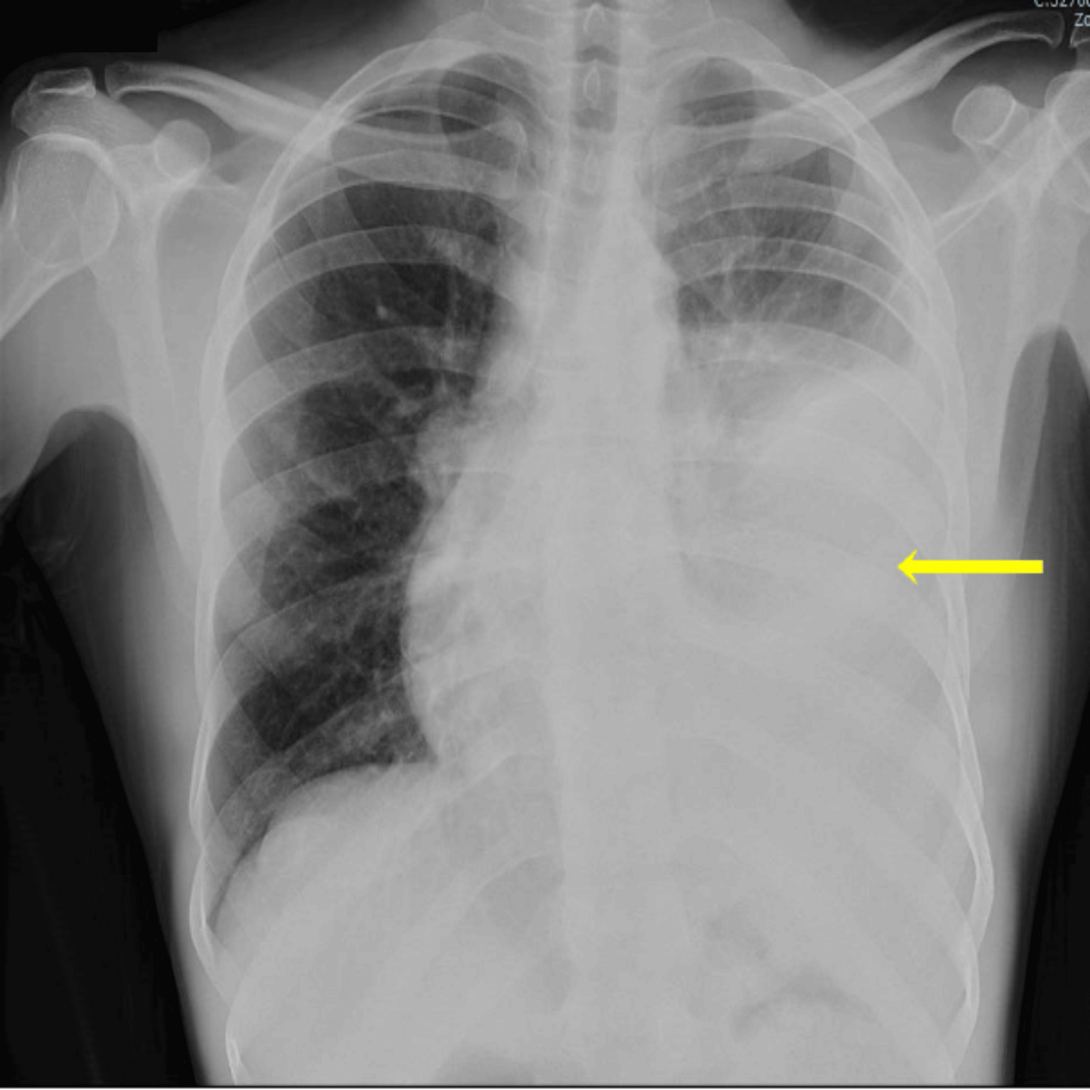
Chest radiograph on hospital admission showing left-sided pleural effusion (arrow).
